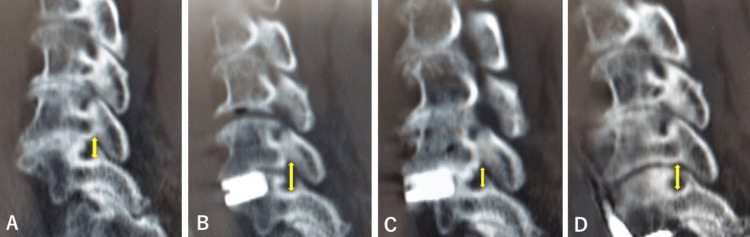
Case 1: Sagittal reconstructed CT scan. Sagittal reconstructed CT scan showing that the diameter of the right C5/6 intervertebral foramen (⇕) was 7.0 mm before the first operation (A), 11.0 mm after the first operation (B), 4.0 mm before the second operation (C), and 9.2 mm after the second operation (D).
